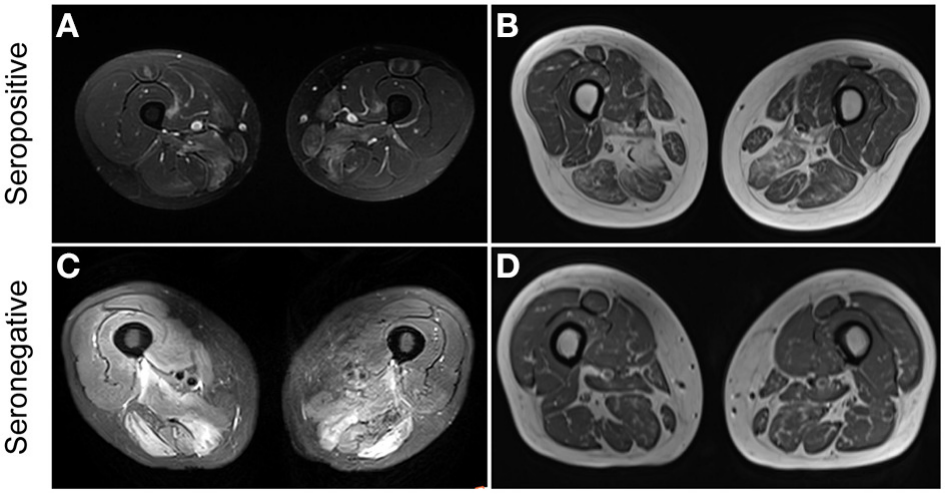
Representative thigh muscle MRI of seropositive and seronegative immune-mediated necrotizing myopathy (IMNM). (A) High signal intensity on MRI STIR images in a patient with seropositive IMNM. (B) Muscle atrophy and fatty replacement on MRI T1 images in a patient with seropositive IMNM. (C) Diffuse increased signals on MRI T2 images in a patient with seronegative IMNM. (D) Muscle atrophy and fatty replacement on MRI T1 images in a patient with seronegative IMNM.
